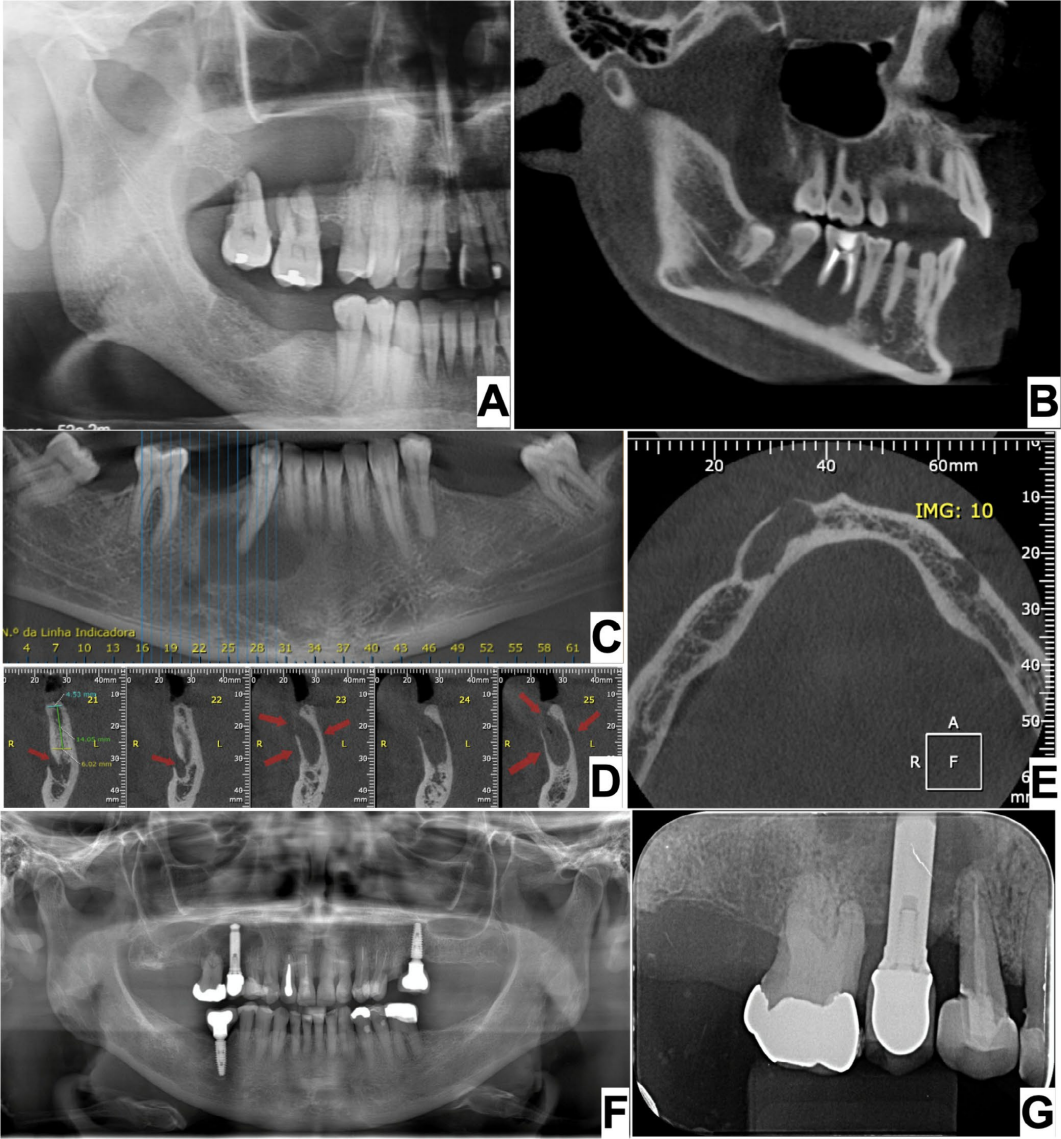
Radiographic features of ANSPNL of some included cases. Metastasis: (A) A panoramic radiograph showing radiolucent, unilocular and well‐defined lesion in the upper right molar region. Ewing sarcoma: (B) A tomography showing a hypodense, unilocular and well‐defined lesion in lower left molar region. Squamous cell carcinoma (SCC): (C) Cone beam computed tomography panoramic view revealing a hypodense, unilocular lesion with a well‐defined/punched‐out margin between the right lateral incisive and premolar. (D) Sagittal view. The red arrows in the images indicate the sites of cortical bone perforation. (E) Coronal view revealing a hypodense lesion with no perforation of buccal cortical bone. Langerhans cell Histiocytosis (LCH): (F) Panoramic radiograph showing a radiolucent lesion in the upper right premolar region. (G) A periapical radiograph of the same patient reveals a radiolucent, unilocular and well‐defined lesion.
